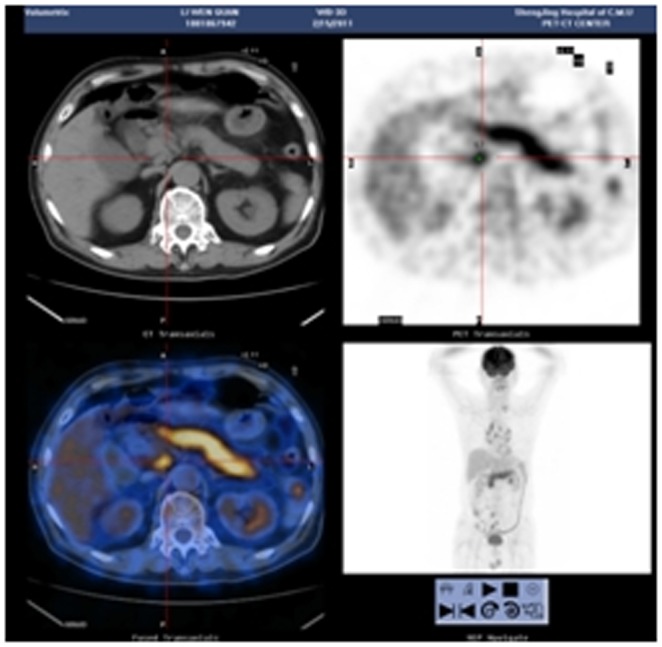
PET-CT revealing swelling and pencil sharp of pancreas with enlargement of peripancreatic lymph nodes.
